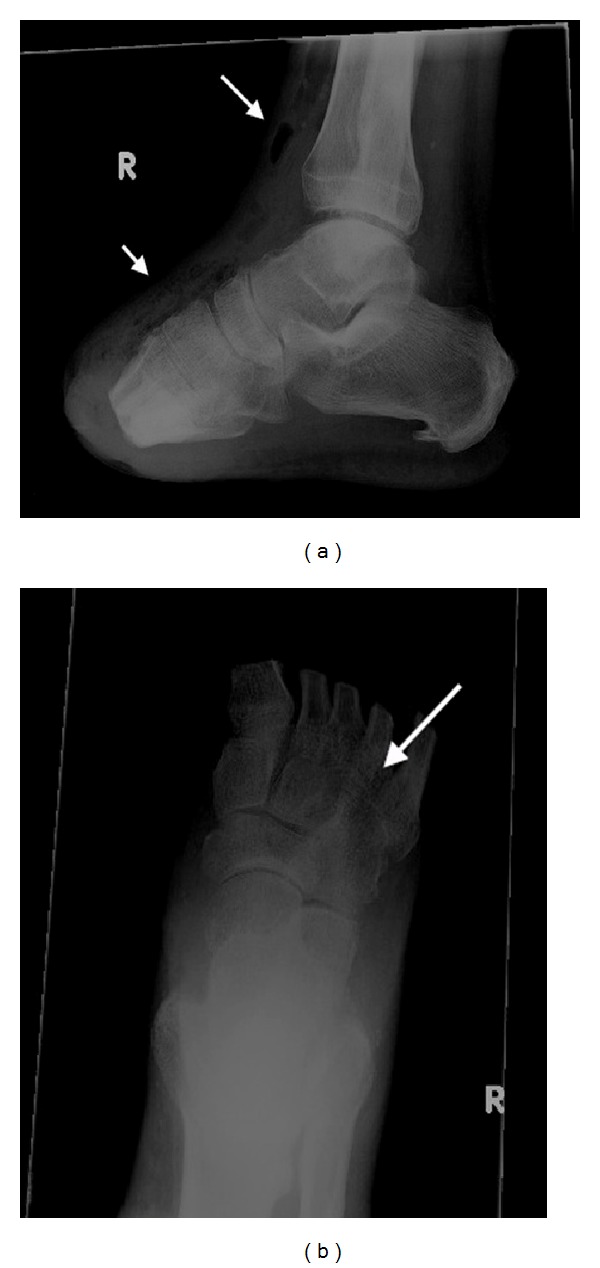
Right foot radiographs reveal extensive soft tissue gas in the dorsum of the foot ((a), short arrow) and anterior to the distal tibia ((a), long arrow). Anteroposterior view demonstrates discrete radiolucencies in the metatarsals remnants ((b), arrow) and midtarsal bones. Previous transmetatarsal amputation is noted.
